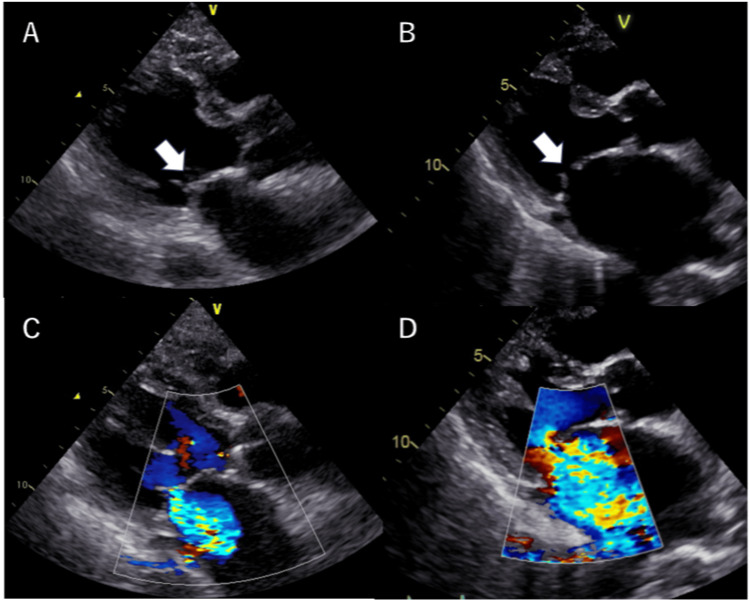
Parasternal long-axis transthoracic echocardiographic views comparing the period between episodes and the acute episode. A: Between episodes (color Doppler off), showing preserved mitral leaflet coaptation (arrows). B: During an acute episode (color Doppler off), demonstrating complete loss of coaptation between the anterior and posterior mitral leaflets (arrows). C: Between episodes (color Doppler on), showing only mild mitral regurgitation (MR). D: During an acute episode (color Doppler on), revealing severe MR with a dagger-shaped jet. MR, mitral regurgitation.
